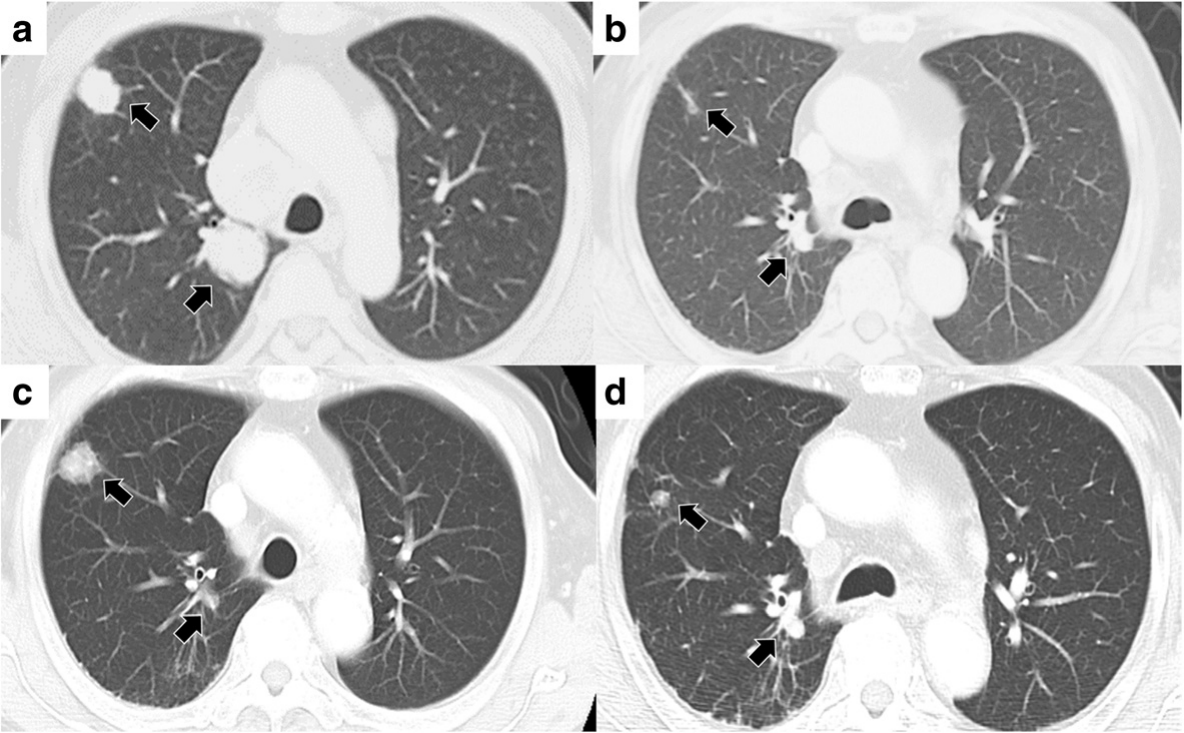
a Computed tomographic scan taken before initiation of treatment with sorafenib reveals multiple pulmonary metastatic lesions (*arrows*). b Computed tomographic scan obtained 1 year after initiation of sorafenib reveals tumor regression (*arrows*) of 70.1 % according to Response Evaluation Criteria in Solid Tumors. c Computed tomographic scan taken 8 months after sorafenib therapy was stopped reveals progression of pulmonary metastases (*arrows*). d Pulmonary metastases (*arrows*) remained stable 11 years after initiation of treatment with sorafenib
